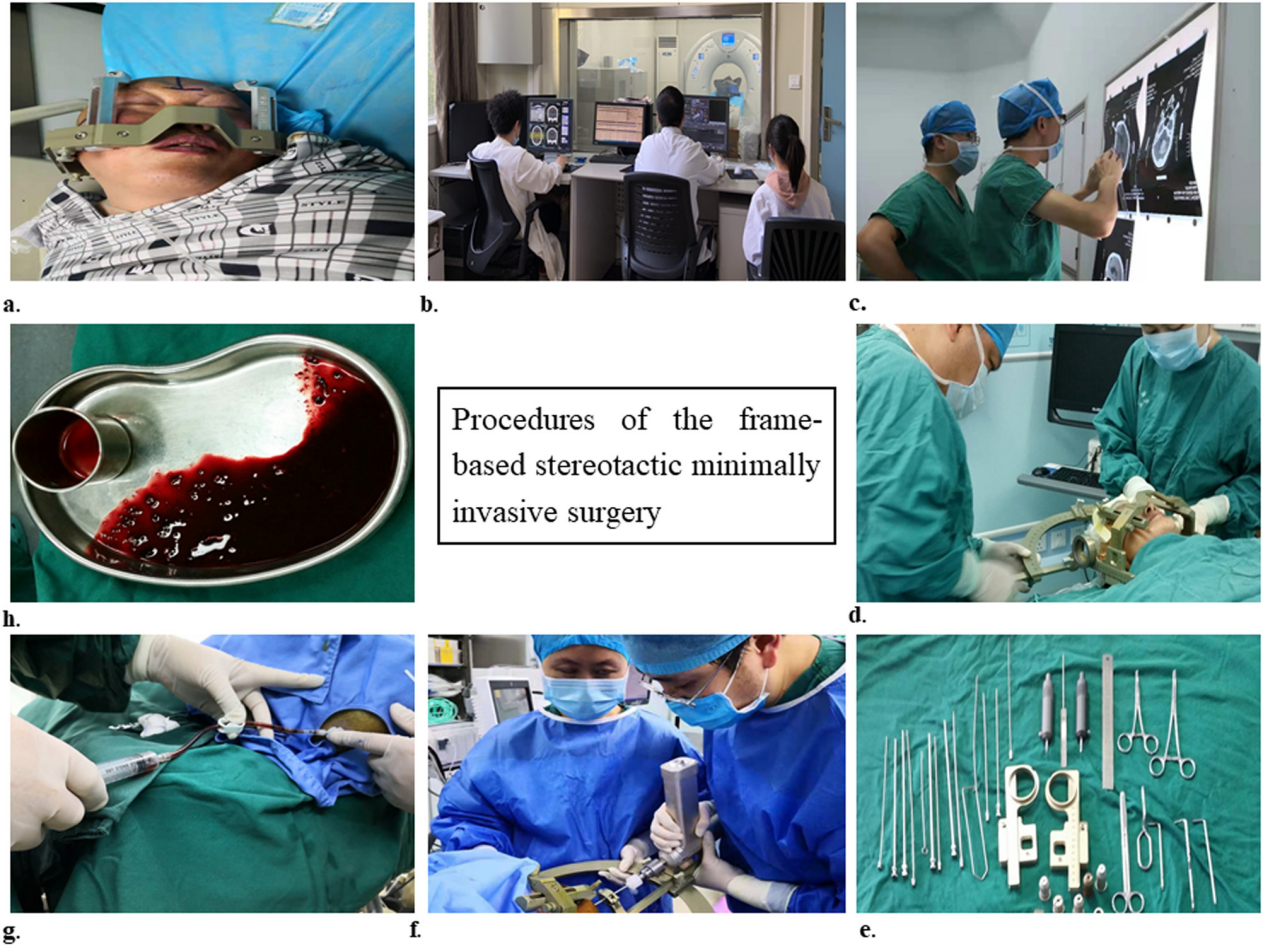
Procedures for the stereotactic minimally invasive surgery. A positioning headframe was fixed on the head first and then the patient was transferred to scan CT for figuring out the coordinates (a–c). Subsequently, the arc frame and guider were fixed to the positioning headframe and a transcranial puncture needle was inserted (d–f). Finally, the liquid part of the ICH was aspirated out (g and h). The puncture needle set and the drainage system were composed of a needle guard, a metal needle core, a plastic needle core, and a plastic drainage tube.
